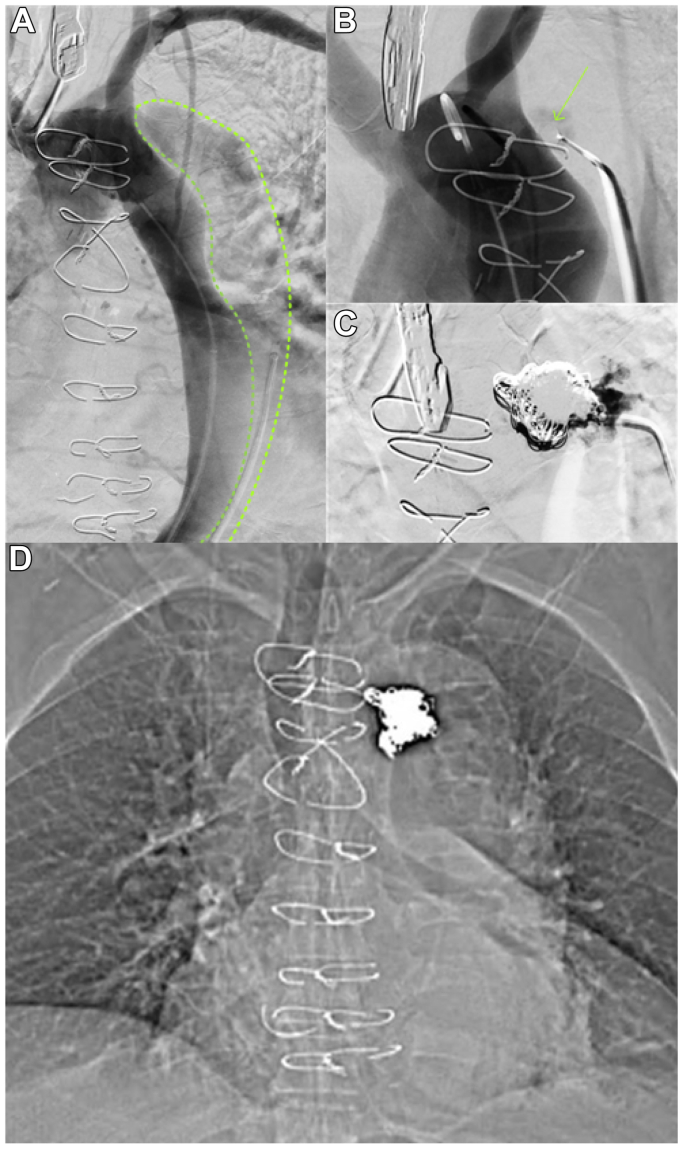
Procedural imaging of stentless thoracic endovascular aortic repair (TEVAR). (A) Selective angiogram via a pigtail catheter within the true lumen shows communication to the false lumen (*green dashes*) before repair. (B) Selective angiogram via a pigtail catheter within the true lumen shows reduced antegrade perfusion into the false lumen after placement of the vascular plug (*green arrow*). (C) Selective angiogram via the Rim catheter within the false lumen shows no communication between the lumens following placement of embolization coils. (D) Coronal unenhanced computed tomography (CT) scan demonstrating positioning of the vascular plug and embolization coil pack.
